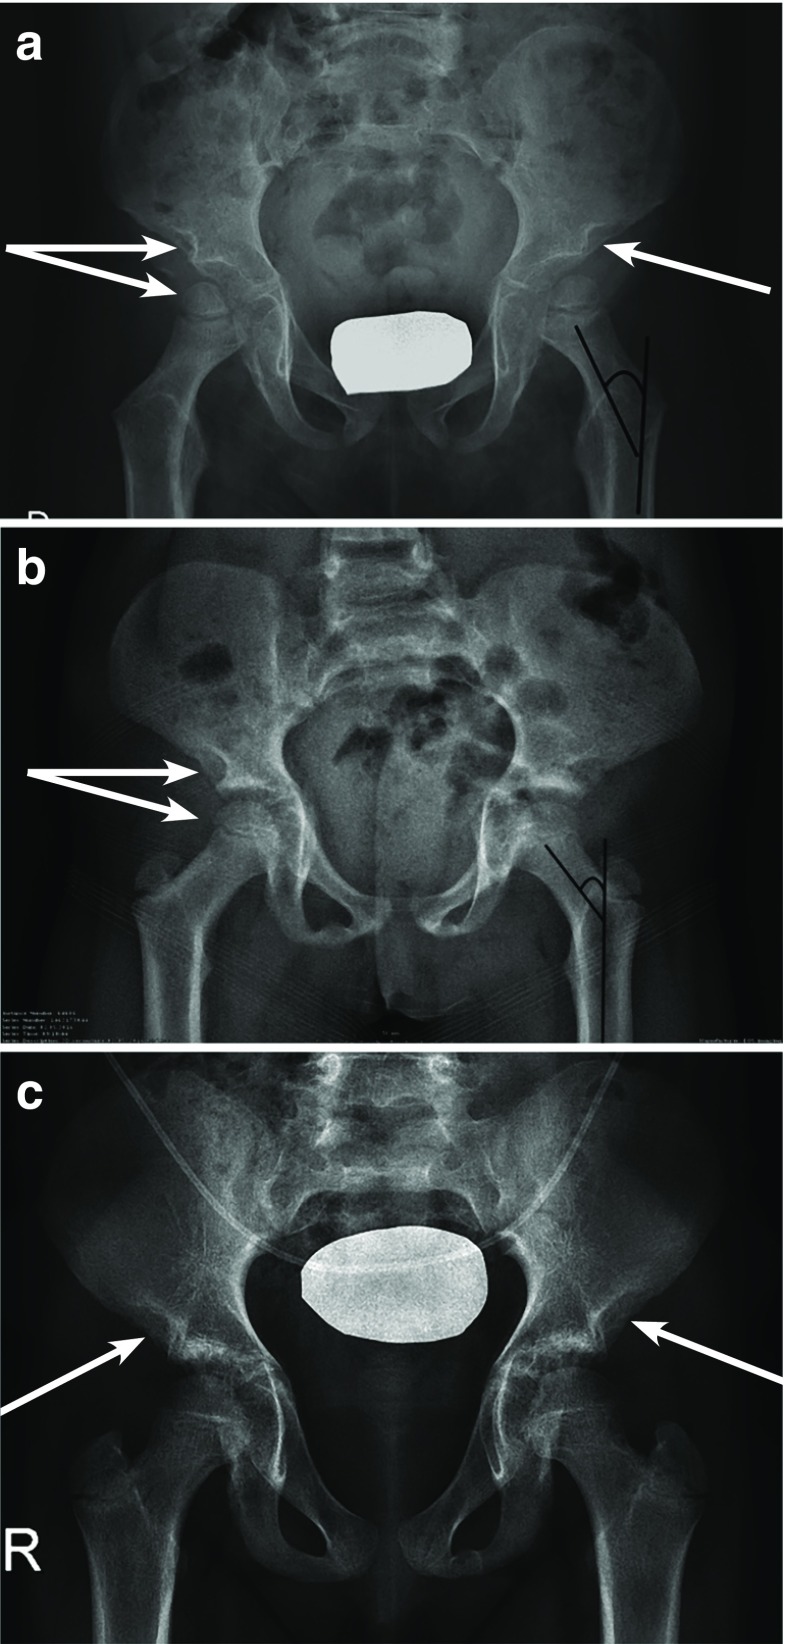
Frontal radiographic image of the pelvis in two children with attenuated MPS I (a 4-year-old (a) and an 8-year-old (b)), the anomalies are similar and associated with long and narrow femoral neck, a superior femoral epiphyses given the short stature, a relative coxa valga, a moderate acetabular dysplasia, and poorly developed acetabulum. Similarly, in a third child (age 12 years), all the anomalies mentioned are much more attenuated, with the exception of the characteristic of acetabulum which is noted in all radiographic images of attenuated MPS I (c)
